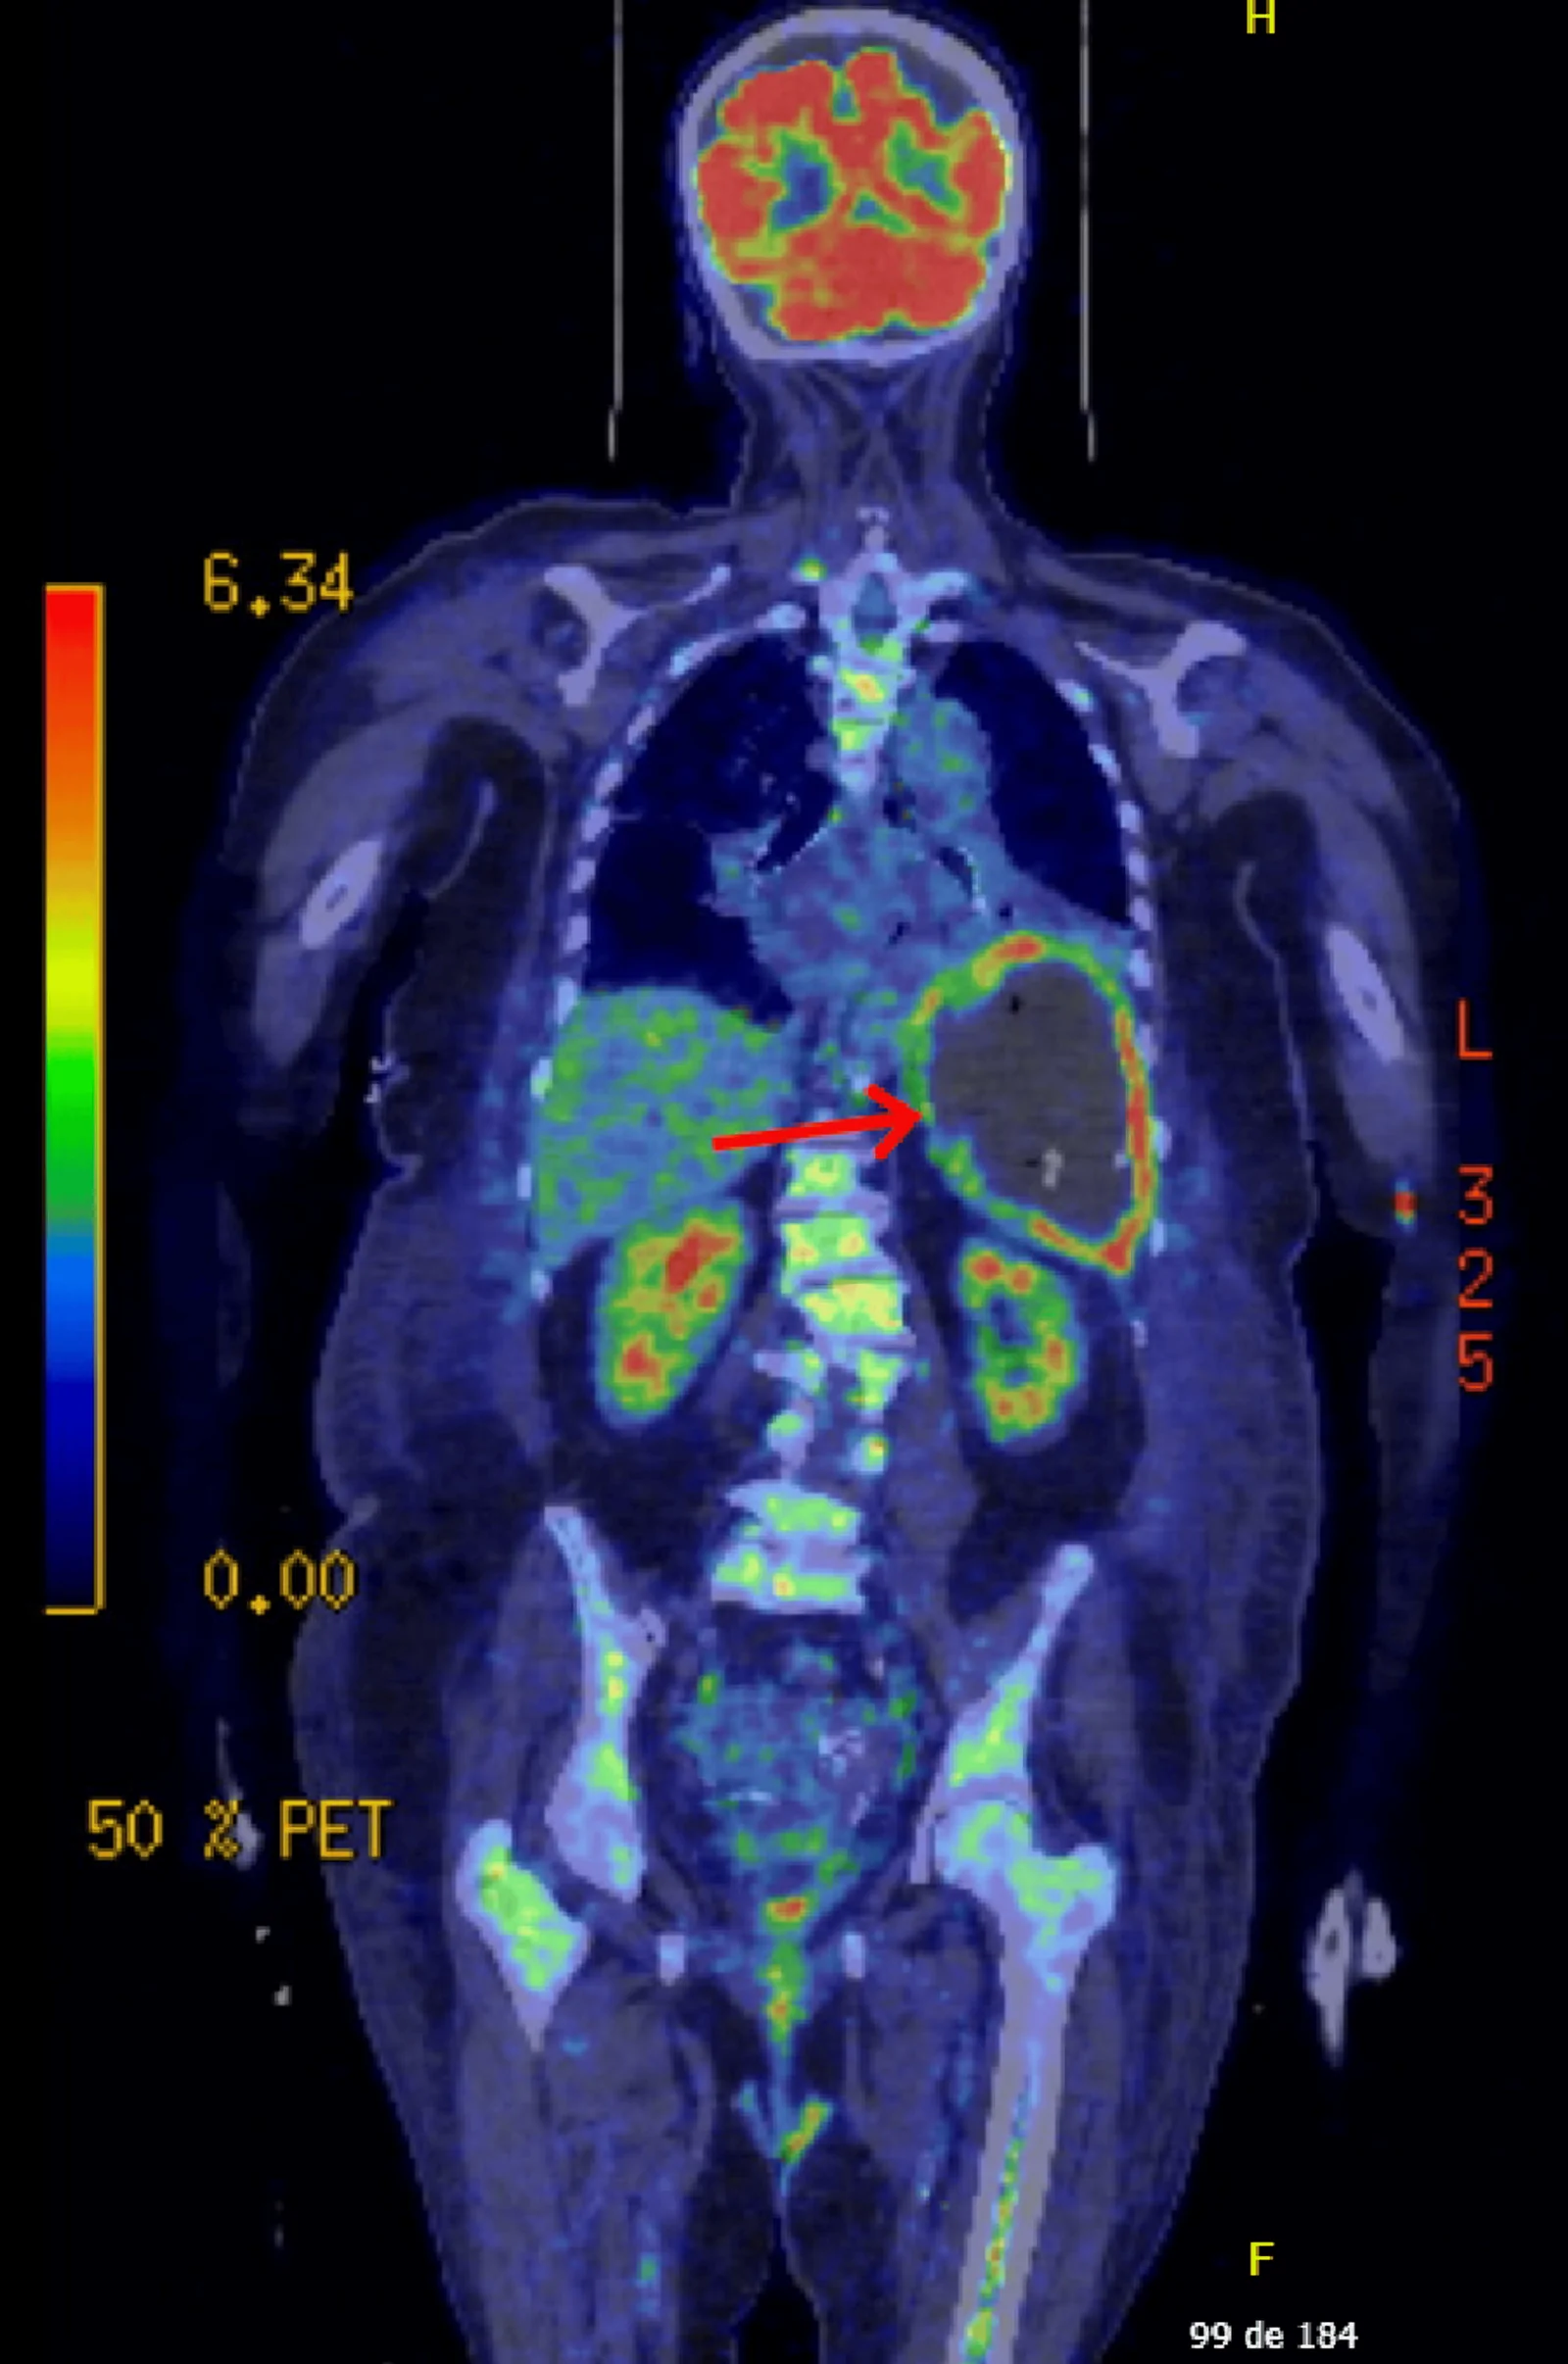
Coronal section of the PET-CT; splenic abscess with hypermetabolic walls indicated by an arrow
